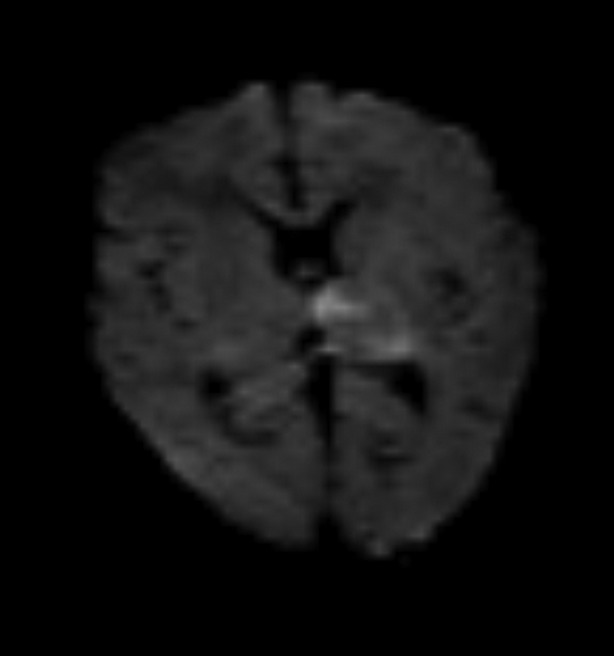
Magnetic resonance imaging on day two. Axial diffusion-weighted imaging demonstrating restricted diffusion in the left thalamus and left internal capsule.
